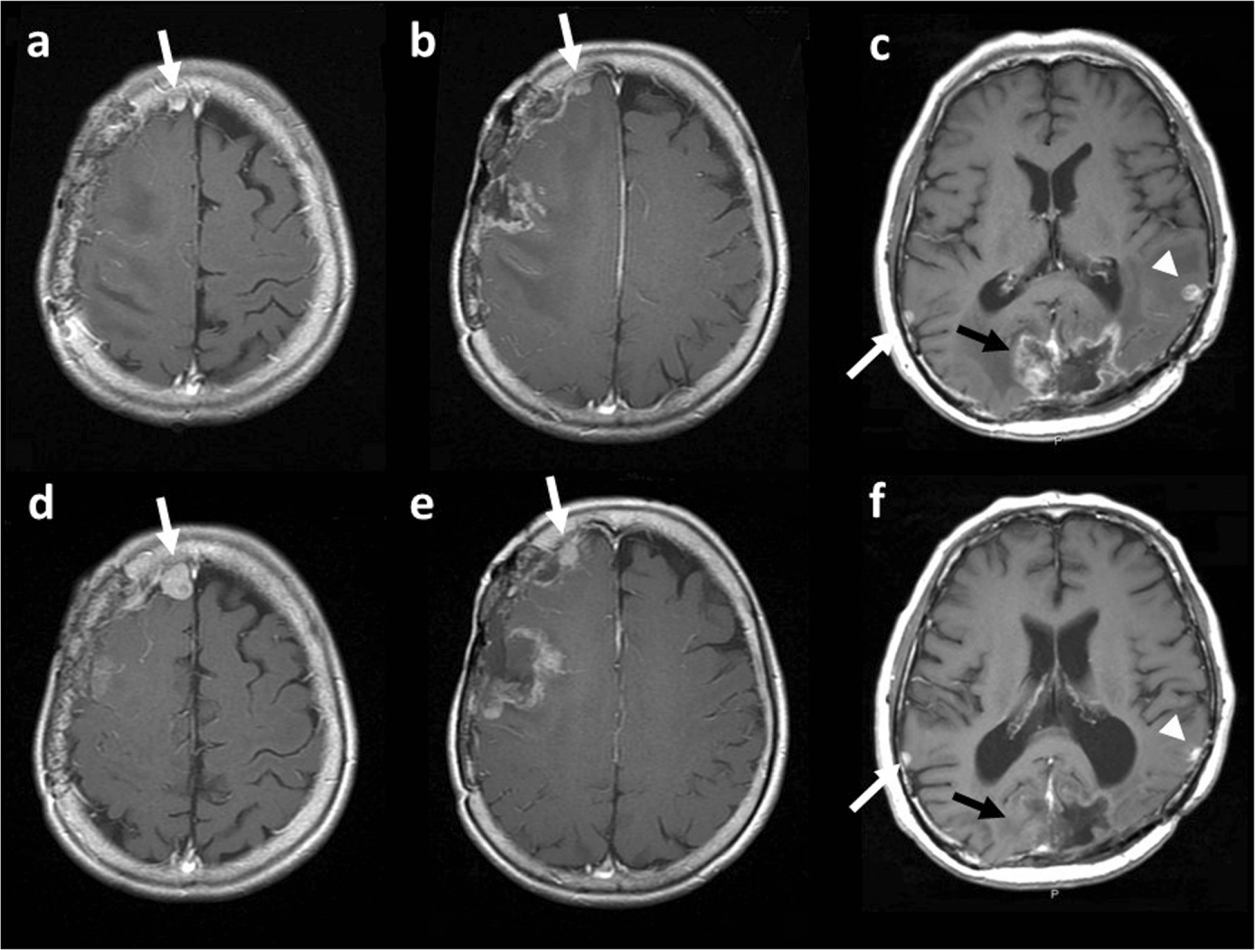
MR images before and after bevacizumab in Case 1 (before, a, b; after, d, e) and Case 2 (before, c; after, f). There were three non-irradiated tumors (a, white arrow: tumor 1; b, white arrow: tumor 2; c, white arrow: tumor 7). After bevacizumab, both Tumors 1 and 2 showed progression (d, e: arrows). Tumor 7 did not changed after bevacizumab treatment (f: arrow). On the other hand, a post-irradiated tumor decreased after bevacizumab. Tumor 6 decreased to −79 % (c, f: arrowheads). Intraparenchymal radiation necrosis was disappeared (c, f: black arrow)
